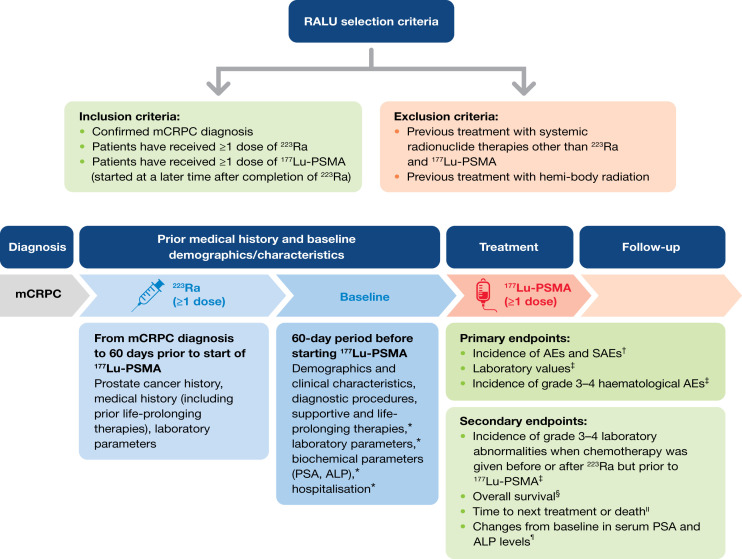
RALU study design. *Data also collected during ^177^Lu-PSMA therapy and during follow-up period after last ^177^Lu-PSMA dose. ^†^Measured from start of ^177^Lu-PSMA therapy up to 30 d after last dose. ^‡^Measured from start of ^177^Lu-PSMA therapy up to 90 d after last dose. ^§^Measured from both start of ^223^Ra and start of ^177^Lu-PSMA. ^‖^Measured from start of ^177^Lu-PSMA. ^¶^Measured during 60-d baseline period and during follow-up. SAE = serious AE.
